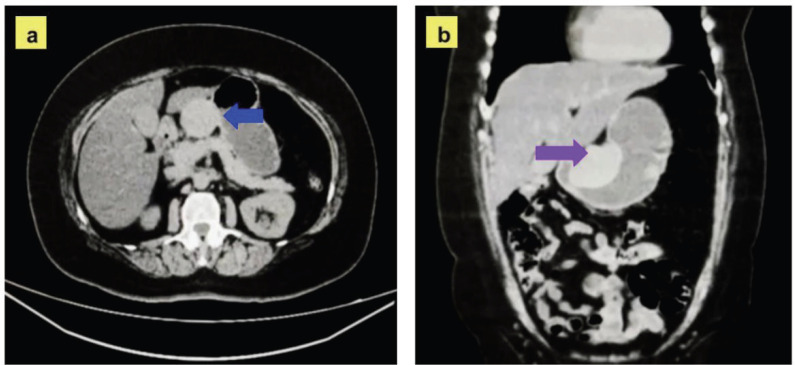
Contrast-enhanced CT with axial and coronal sections. (a): Axial section showing a solid lesion with well-defined borders, located in the lesser curvature of the stomach (blue arrow). The lesion measures approximately 64 × 49 mm, has homogeneous density and no invasion of adjacent structures. (b): Coronal section confirming the location of the lesion in the lesser curvature of the stomach (purple arrow).
